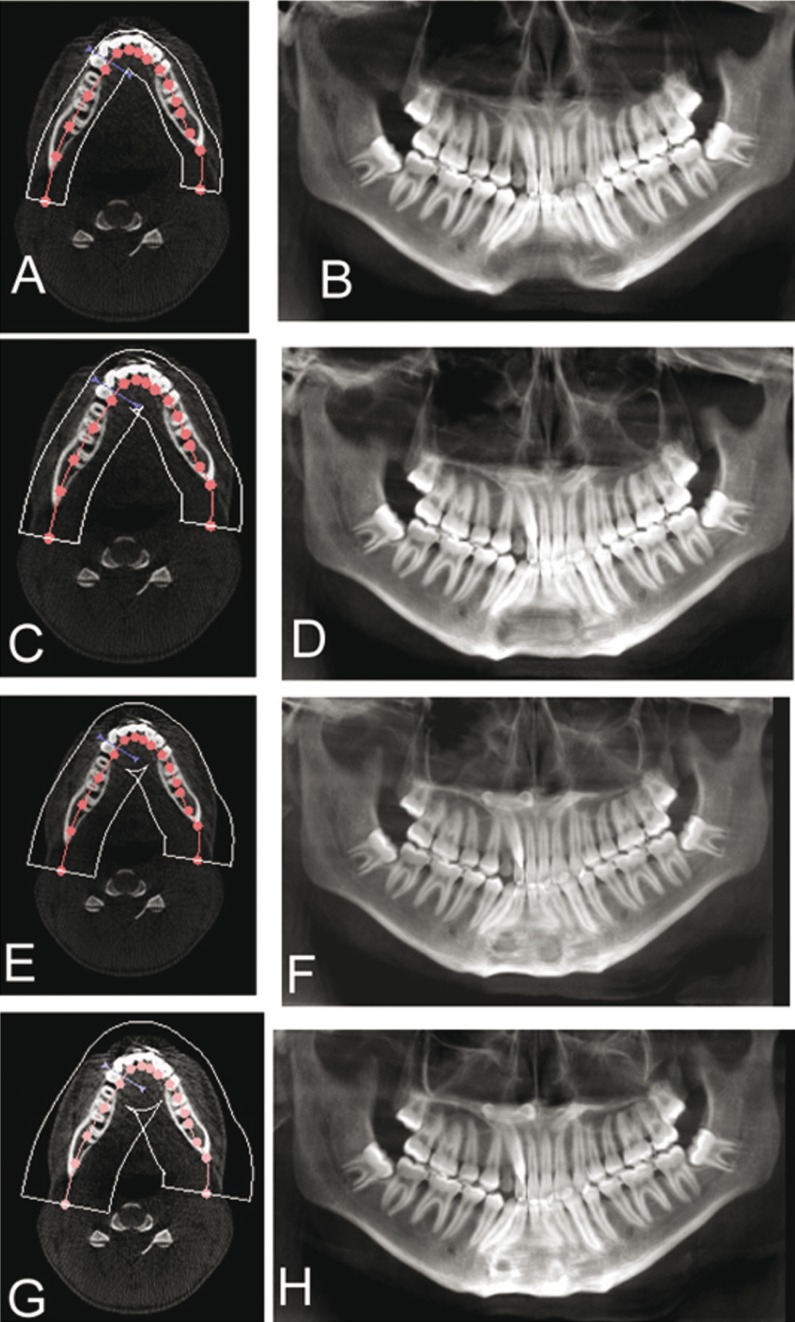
RPR re-constructed from the CBCT with varying trough sizes. Width of the trough: A – 20.1 mm, B (OPG from A); C – 29.7 mm, D (OPG from AC; E – 39.1 mm, F (OPG from E); G – 50.1 mm, H (OPG from H).
